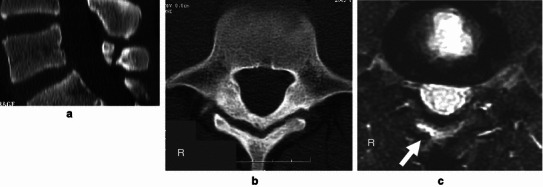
a CT images show a bony defect intralaminally. b MRI shows signal changes along the defect with high intensity on a fat-saturated T2-weighted image
